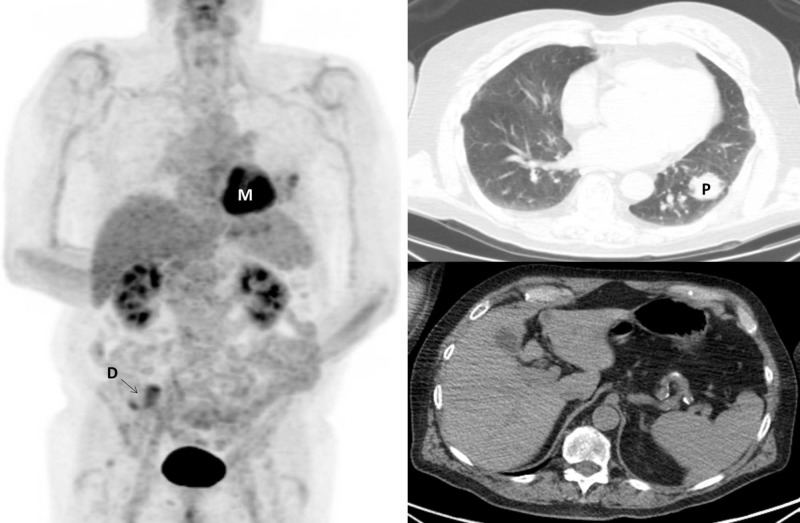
Surveillance imaging at 41 months from diagnosis. PET/CT maximum intensity projection (left) and representative transverse CT chest (right, top) and CT abdomen (right, bottom) images are shown. The myocardium is FDG-avid (M; left) and the patient had mildly avid diverticulosis (D; left). There is no evidence of metabolically active disease. The left lung parenchymal lesions treated with SBRT (P; right, top) are stable in size and morphology (right, top). The initially seen right-sided pulmonary parenchymal nodules have demonstrated stable partial response. The omental mass has radiographically resolved (right, bottom). PET/CT: Positron emission tomography/computed tomography; FDG: Fluorodeoxyglucose; SBRT: Stereotactic body radiation therapy.
